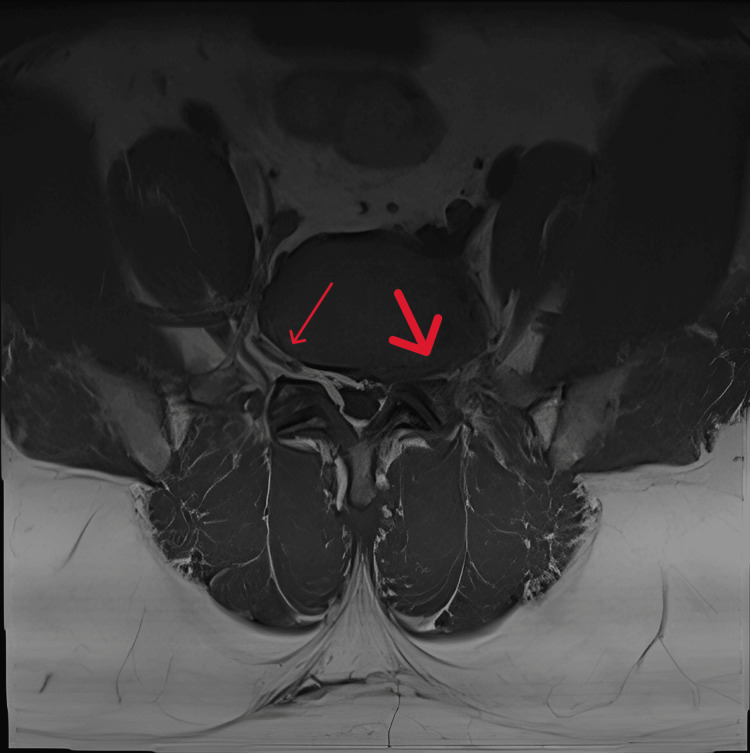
Axial view of T1-weighted MRI images, with a small red arrow indicating no stenosis and a large red arrow indicating extraforaminal stenosis with an absence of perineural fat signal
